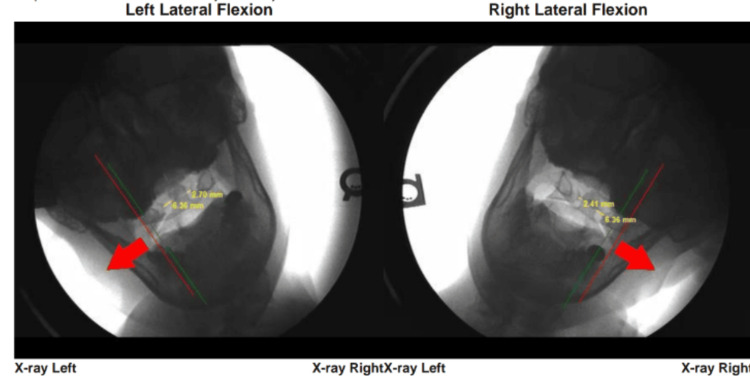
DMX image(s) - 3 Lateral bending of the cervical spine in the AP view demonstrating abnormal overhang of the C1 lateral mass on C2 dynamically when bending the neck laterally from left to right from the neutral position. The red line represents the position of the atlas lateral mass in the side-bending position. The green line represents the position of the axis superior articular process. Shifting of the red line greater than 3.0 mm from the green indicates probable damage to the alar and accessory ligaments DMX: digital motion X-ray
